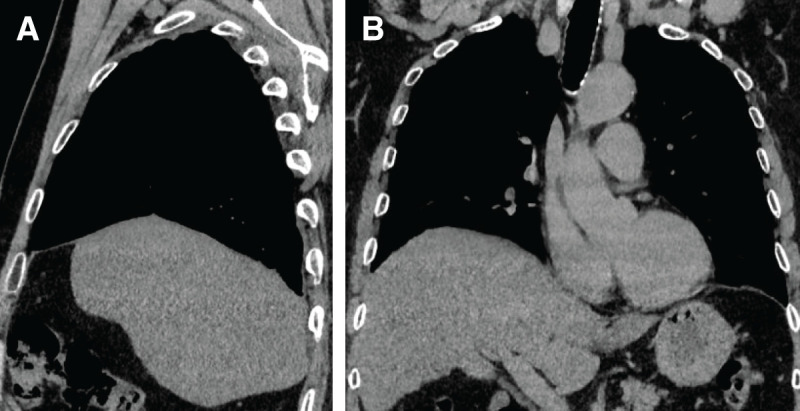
Postoperative CT images. (A, B) CT images 18 months after surgery. The images show that it remains well without evidence of recurrence of intradiaphragmatic abscess.
